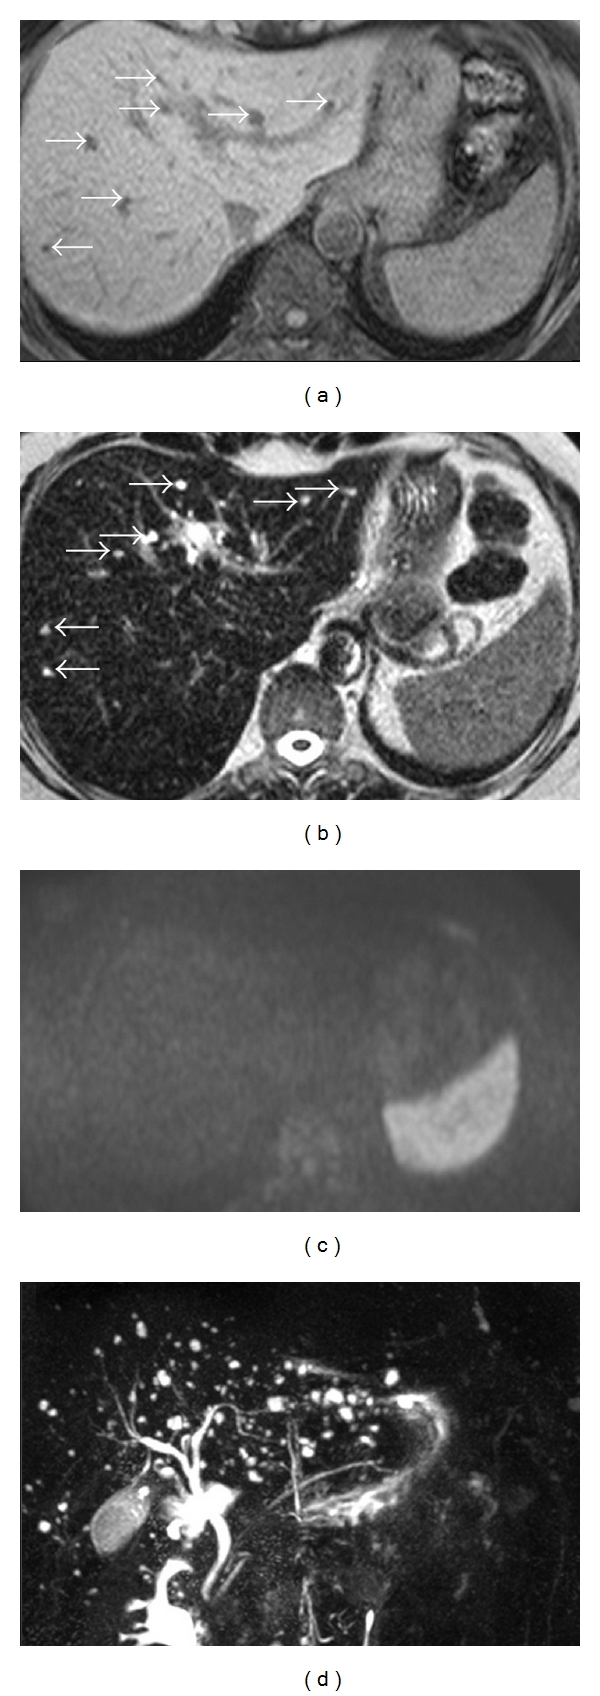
On T1-weighted image of the liver (a), multiple, small, hypointense nodular lesions with slightly irregular contours (white arrows) are demonstrated, which are hyperintense (b) (white arrows) on T2-weighted image. Diffusion-weighted image (c) reveals no restriction of diffusion in the liver lesions. MRCP (d) depicts an abundant number of hyperintense small lesions allover the liver which show no association with the biliary system. The widths and contours of intrahepatic and extrahepatic bile ducts and pancreatic canal are normal.
